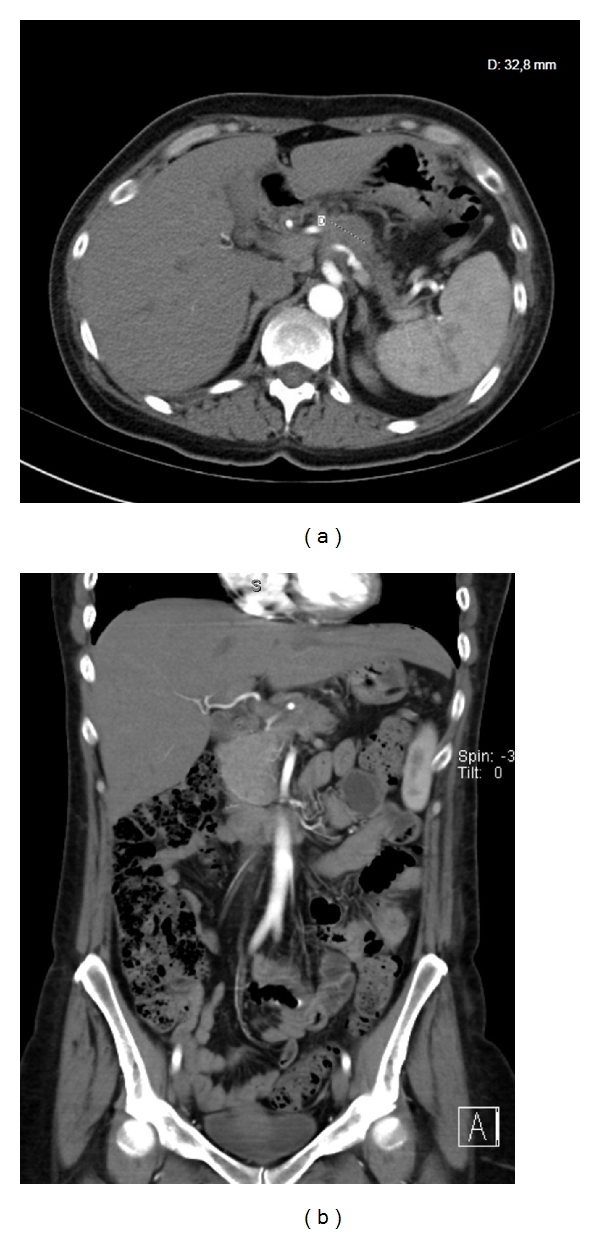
(a) Preoperative arterial phase contrast-enhanced CT scan showing a 3.3 cm lesion in the body of the pancreas. (b) The coronary slice displays tumor infiltration of the celiac trunk, and the superior mesenteric artery is not involved.
